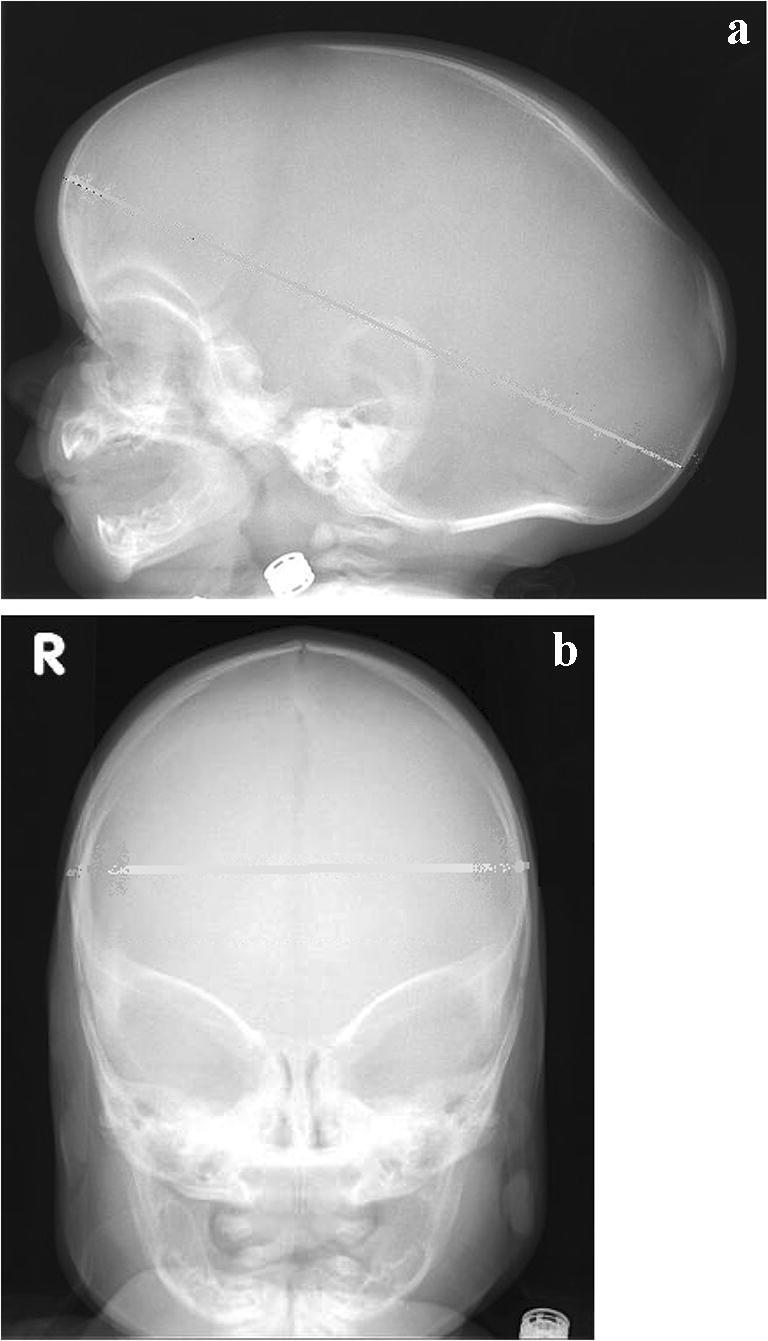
Conventional skull X-ray for visual score. This patient had a visual score of 12. a Lateral view. b Anterior-posterior view
